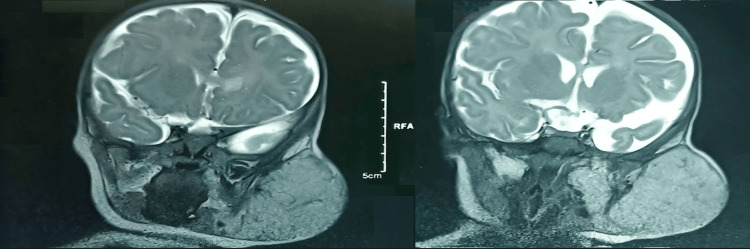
Cervical MRI showing a parotid hemangioma.
